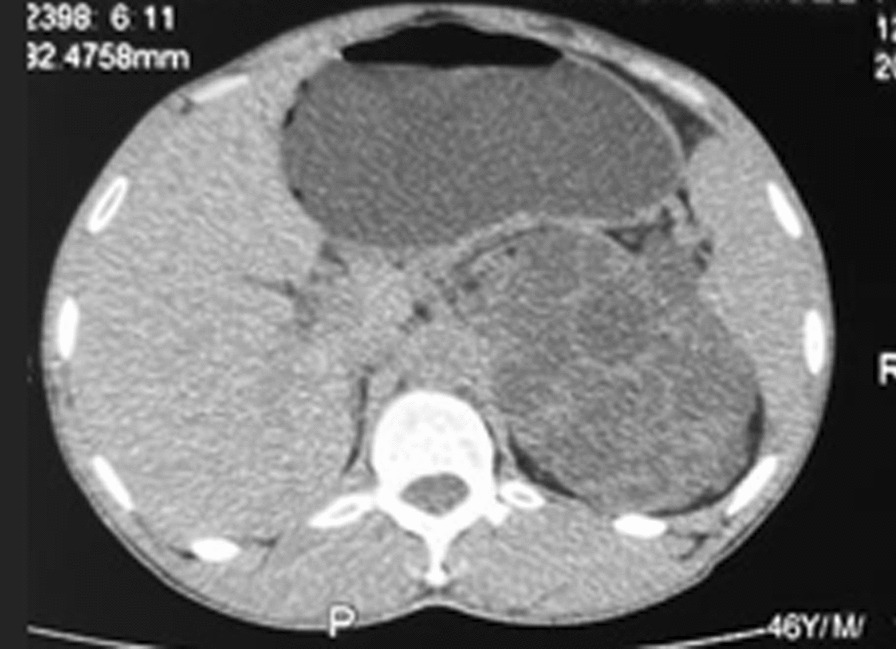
Left orthotopic polycystic kidney disease on CT—Scan (transverse view)
